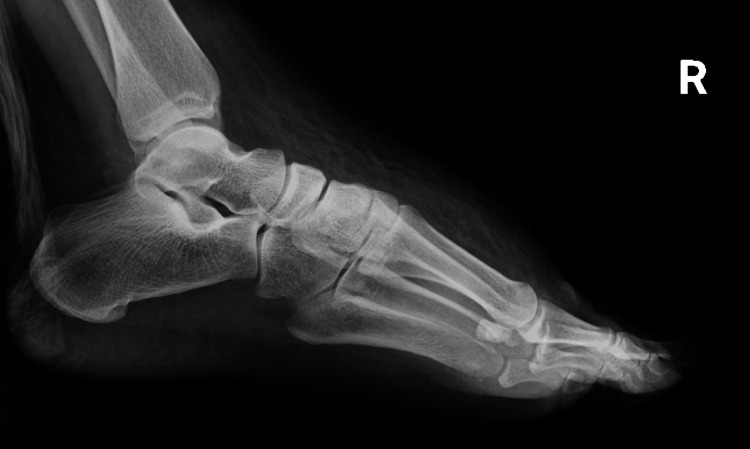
X-ray of the right foot demonstrate severe soft tissue edema without subcutaneous air or osseous changes, consistent with cellulitis.
